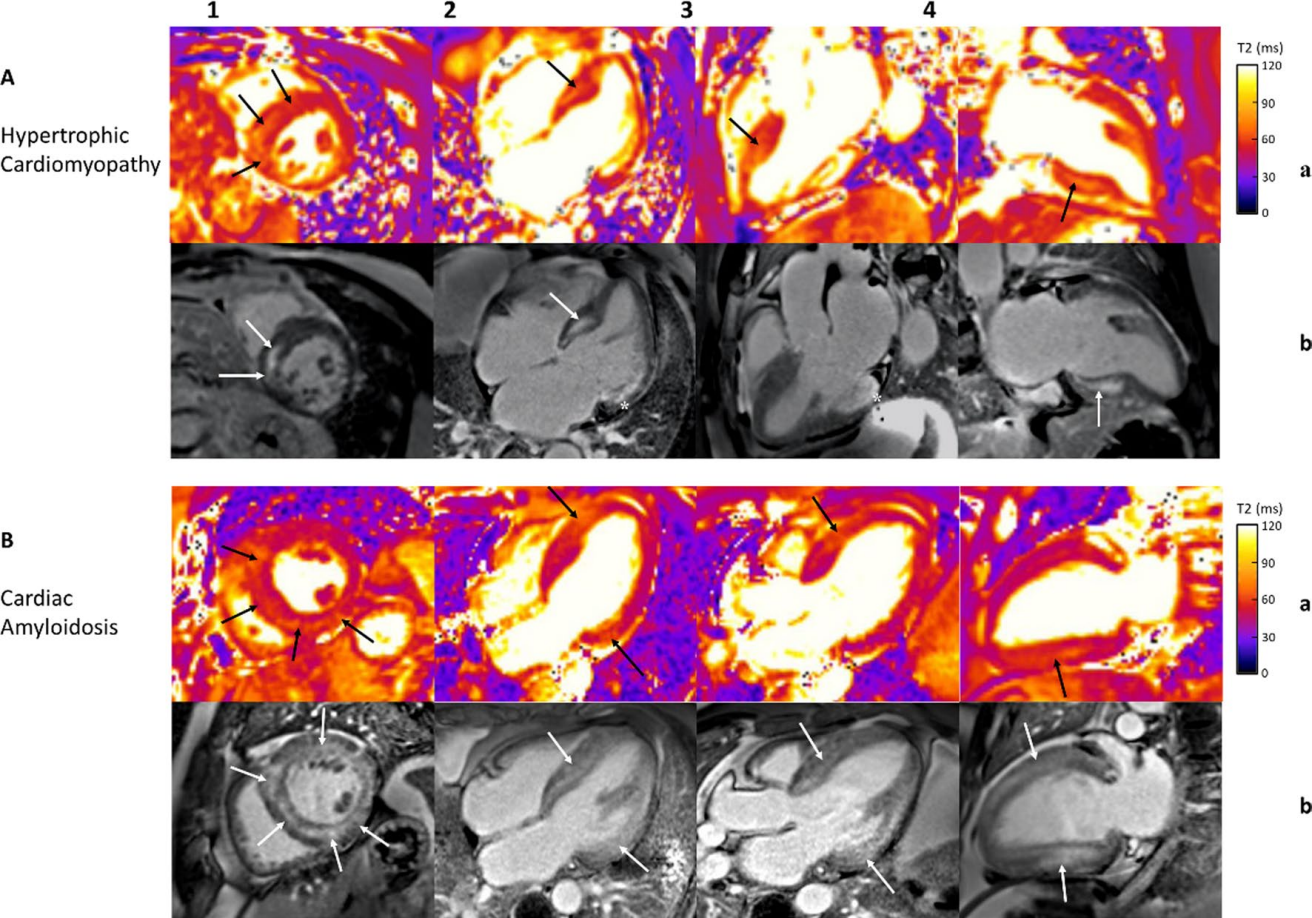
Cardiovascular Magnetic Resonance Imaging in Patients with Increased Left Ventricular Wall Thickness. Panel a: T2 mapping; Panel b: late gadolinium enhancement imaging. Panel 1—midmyocardial short axis view; 2—horizontal long axis view; 3—three chamber long axis view; 4—vertical long axis view. A Patient with hypertrophic cardiomyopathy with myocardial edema/inflammation in the septal and inferior walls (Panel a1–a4; black arrows) and prominent midmyocardial nonischemic fibrosis in the basal to mid septal and inferior walls (Panel b1–b2, b4; white arrows). In addition, there is infarct scar along the basal to mid lateral walls (Panel b2–b3; asterisks). B Patient with cardiac amyloidosis with myocardial edema/inflammation in the septal, inferior and lateral walls (Panel a1–a4; black arrows) with diffuse late gadolinium enhancement throughout the left ventricle (Panel b1–b4; white arrows). T2-prepared bSSFP pulse sequence with the use of rectangular T2 preparation pulse utilized to acquire T2 maps during diastole (1.5 T MAGNETOM Sola, Siemens Healthineers)
